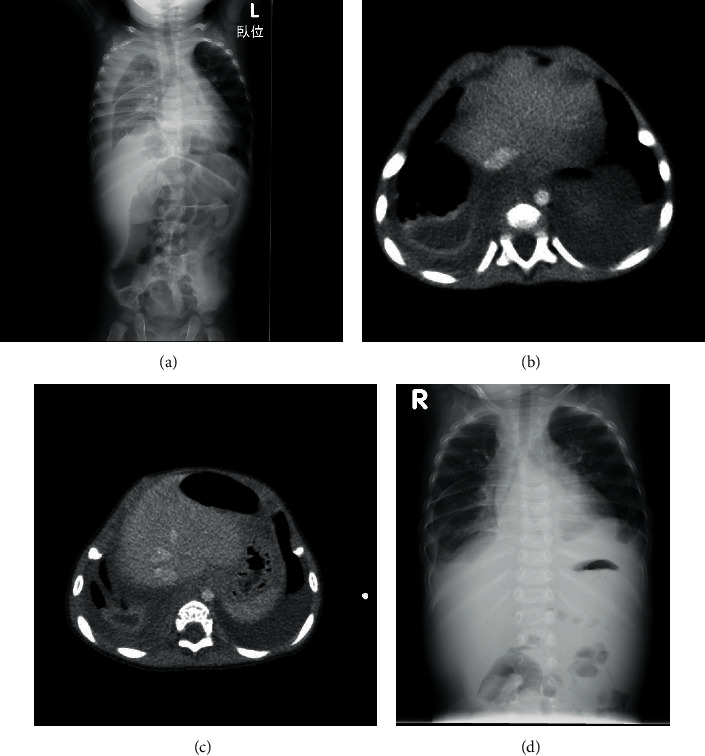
(a) Chest radiograph on admission. (b) Contrast-enhanced CT on the 18th and (c) 55th day of admission. (d) Chest radiograph at discharge.
